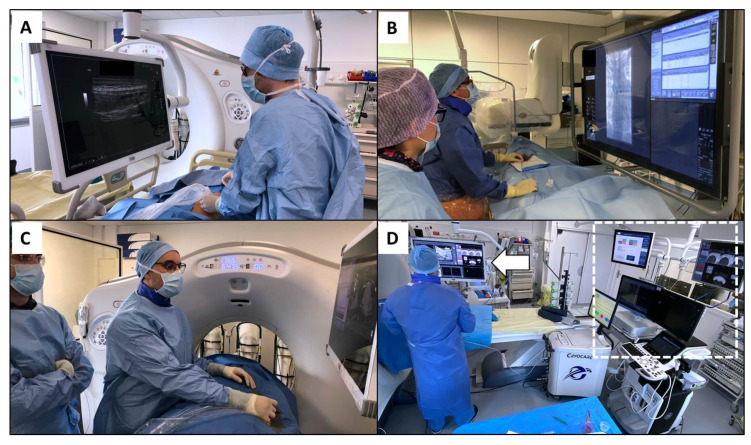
During procedures performed under ultrasound (A), X-rays (B), or under computed tomography scan (C), the screens should be placed in front of the operator to allow a downward viewing angle of 10–15°, which improves the position of the operator’s cervical spine during the whole procedure. Ceiling-mounted monitors can be placed in a wide variety of positions while gathering all the information needed by the operator, including the electromagnetic navigation system, and reducing the congestion of space (D, arrow). Head and body movements used to navigate and search among the screens of all the systems required are therefore limited (to the right, as shown by the dashed line).
